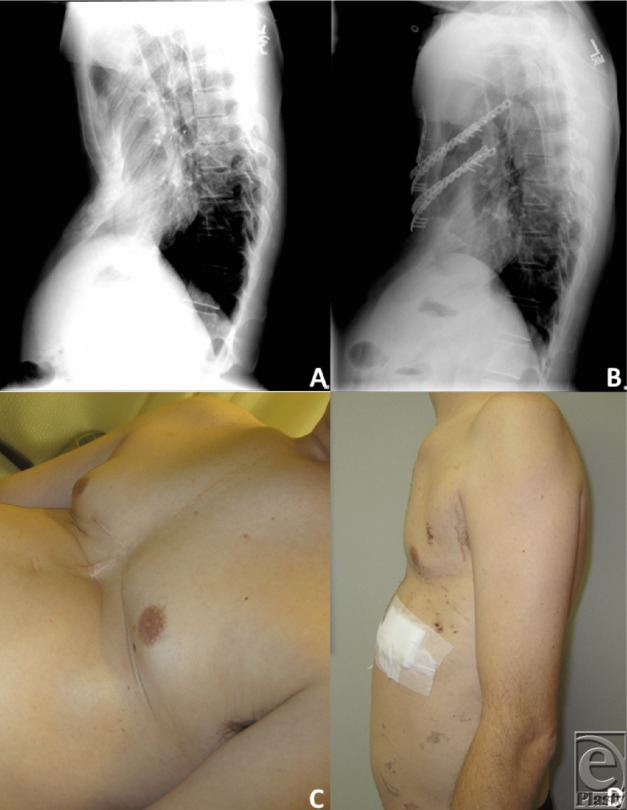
Preoperative (*a*) and postoperative (*b*) roentgenograms, showing change in concavity with the ladder plate and artificial ribs in place. Preoperative (*c*) and postoperative (*d*) patient images showing cosmetic and structural improvement.
